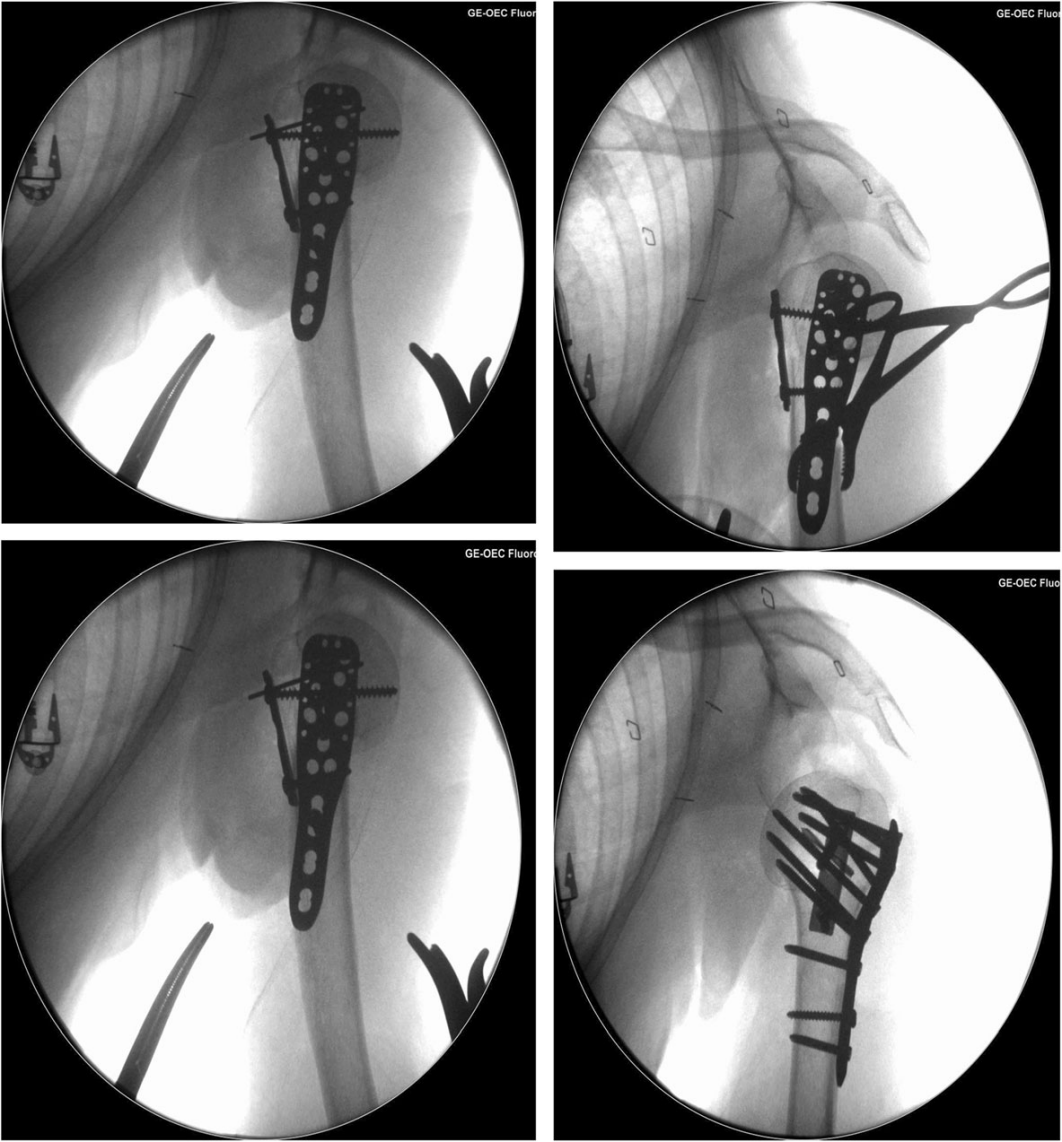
Intra-operative radiographs
